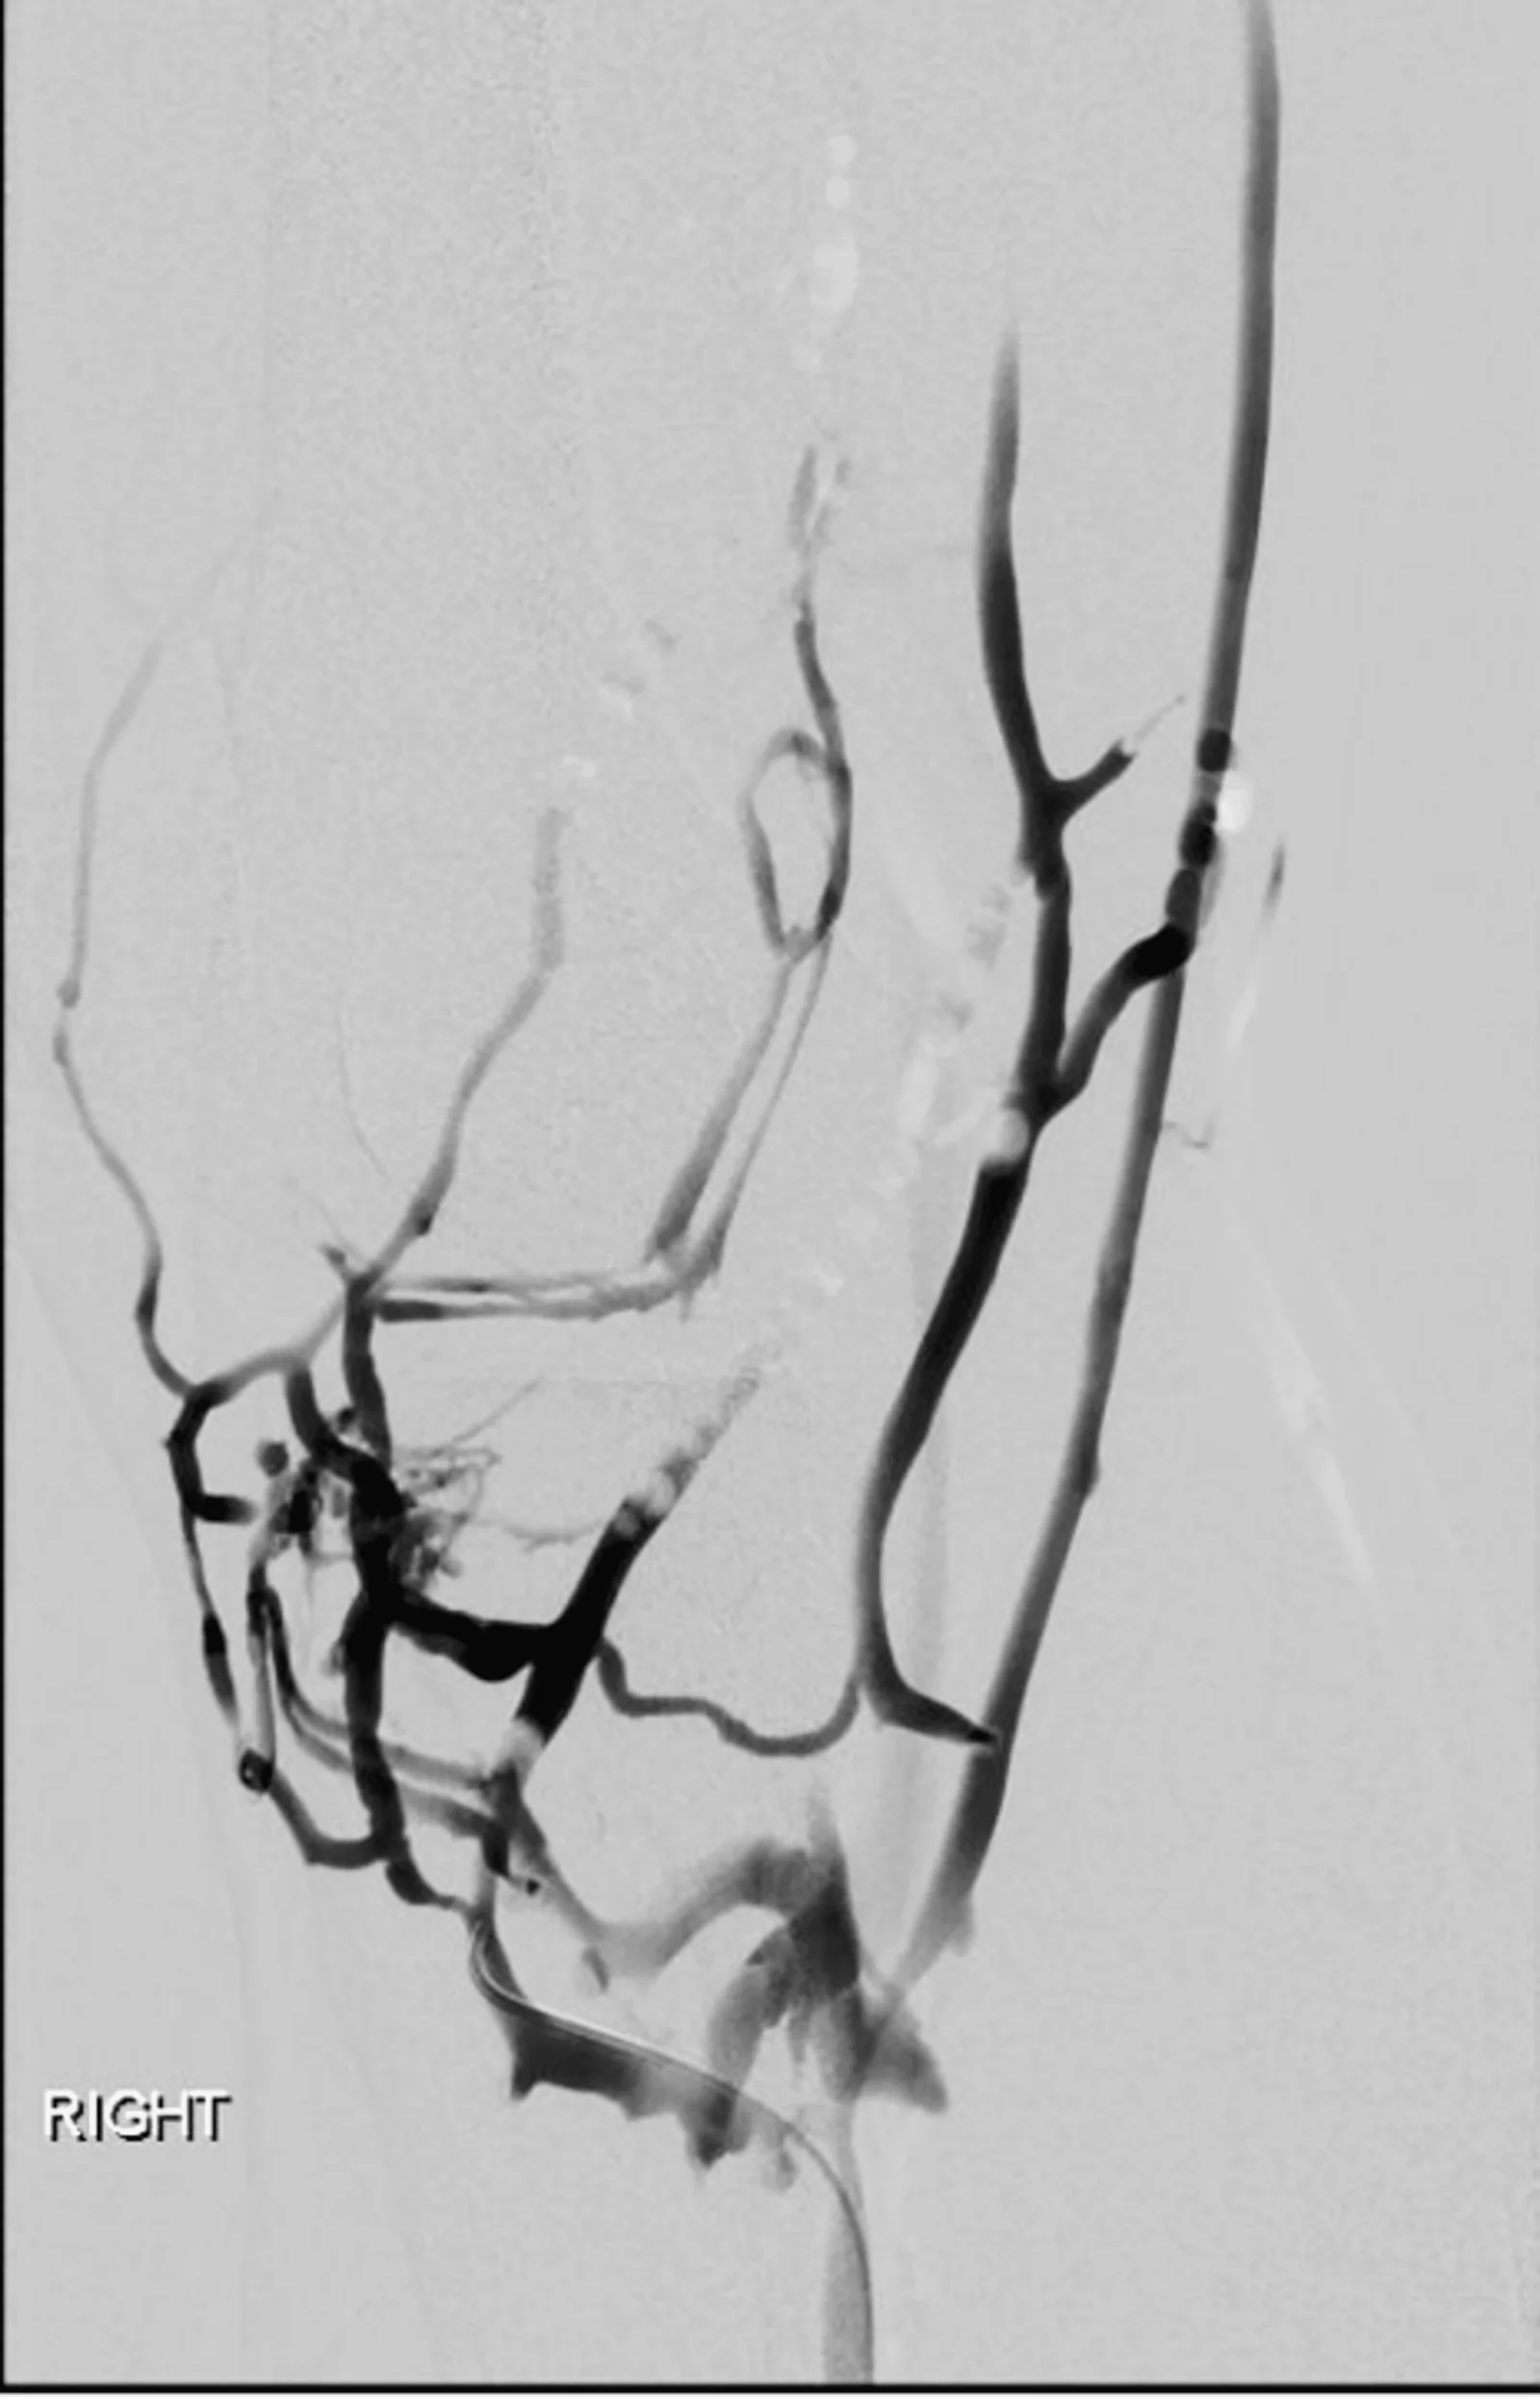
Intraoperative angiogram demonstrating a small tangle of beaded-appearing veins, likely corresponding to the intra-articular venous malformation identified on prior MRI.
